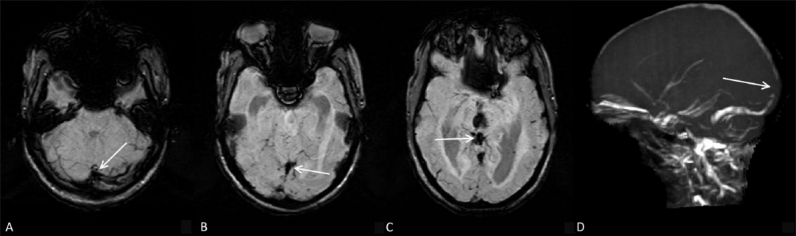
A 30-year-old female with acute onset of headache and altered sensorium. Axial SWI images (A–C) show thrombosis (arrows) at the level of the torcula (A), the straight sinus (B), and the vein of Galen (C), which is confirmed on TOF MRI venography (arrow in D)
